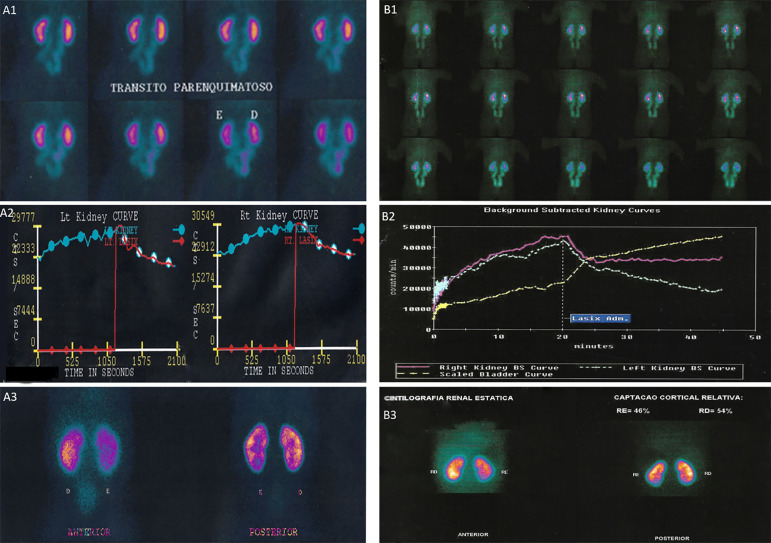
Dynamic and static renal scintigraphy at 5 months of age (A1, A2, A3) and 3 years of age (B1, B2, B3) showing scintigraphic pattern compatible with bilateral hydronephrosis secondary to stasis at the level of the vesicoureteral junction (VUJ) with preservation of kidney function. A1, B1. Dynamic renal scintigraphy showing retention of the radiotracer in the pyelocaliceal system bilaterally (rate of 1 minute/frame). A2, B2. Dynamic renal scintigraphy curves also showing retention of the DTPA marker, even with administration of furosemide, and incomplete drainage of the excretory system, due to partial obstruction of VUJ, considering the slow descending pattern of the renogram curve. A3, B3. Static renal scintigraphy showing preserved kidney function and symmetrical radiotracer expression with relative kidney function, being left kidney of 46% and right kidney of 54%.
